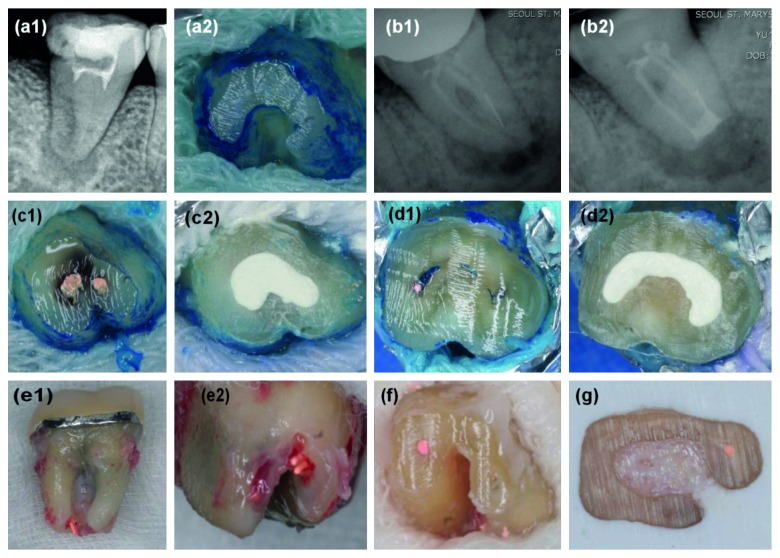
Representative photos of various causes of endodontic failure of C-shaped root canals. (a1) Calcified canal: preoperative periapical radiography. (a2) Root-resected surface of calcified C-shaped root canals. (b1) File separation: preoperative periapical radiography. (b2) Postoperative periapical radiography. (c1) Leaky canal. (c2) Retrograde filling with MTA. (d1) Isthmus connecting mesial and distal canals. (d2) Retrograde filling with MTA. (e1, e2) Overfilling: overextended gutta-percha. (f) Missing canal: only the mesiolingual canal is filled with gutta-percha in the mandibular left second molar. (g) Missing canal: only the palatal canal is filled with gutta-percha in the maxillary right first molar.
